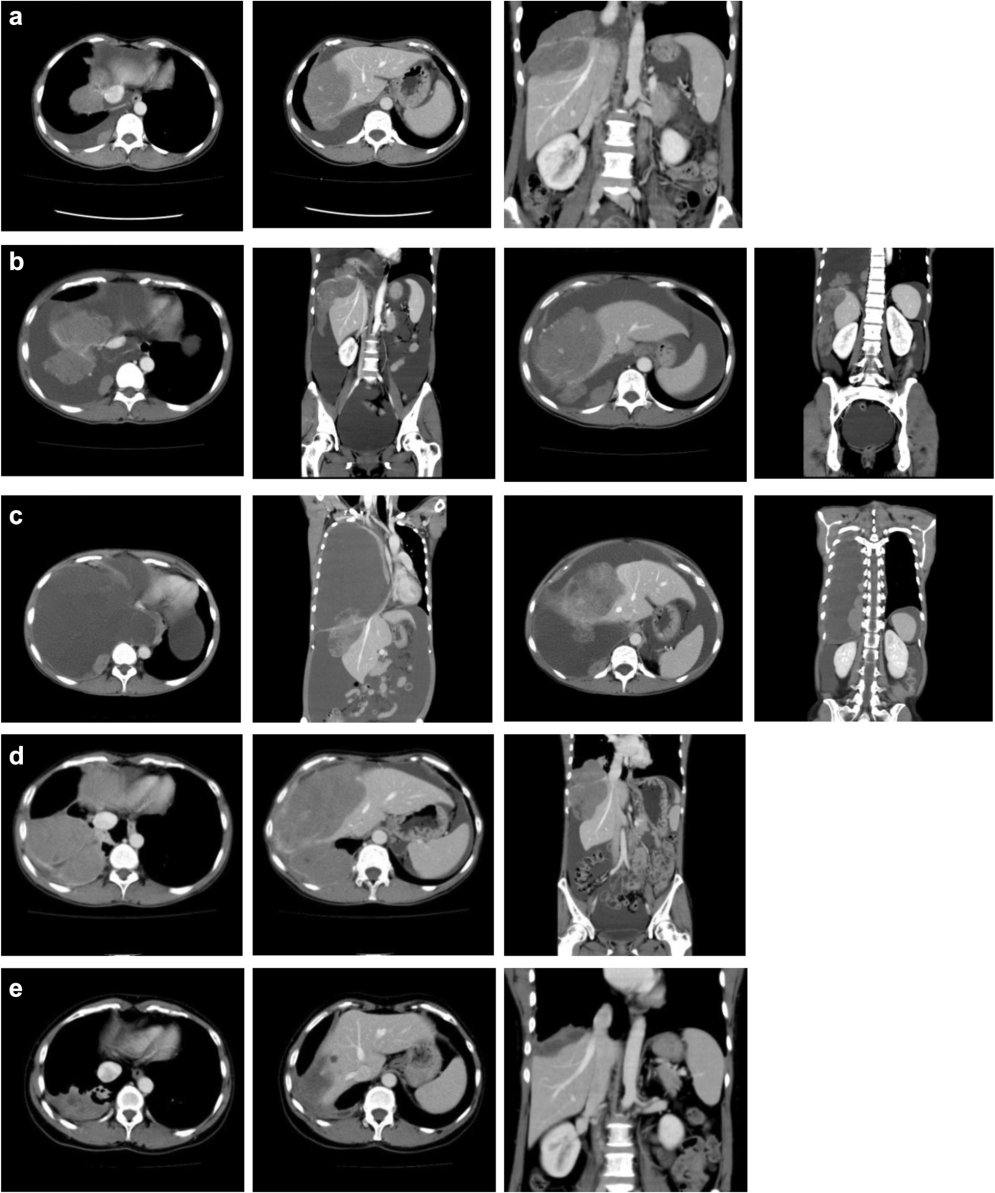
Enhanced computed tomographic scans obtained after tumor recurrence around the right diaphragm and liver surface. a Recurrent tumor found before administering gemcitabine and docetaxel combination chemotherapy. b Bleeding in the abdominal cavity after administering gemcitabine and docetaxel combination chemotherapy. c Four weeks after starting medroxyprogesterone acetate and one week after starting leuprorelin acetate. d Before administering paclitaxel and carboplatin combination chemotherapy and after administering medroxyprogesterone acetate, leuprorelin acetate, and anastrozole. e After administering 18 cycles of paclitaxel and carboplatin combination chemotherapy and medroxyprogesterone acetate, leuprorelin acetate, and anastrozole
